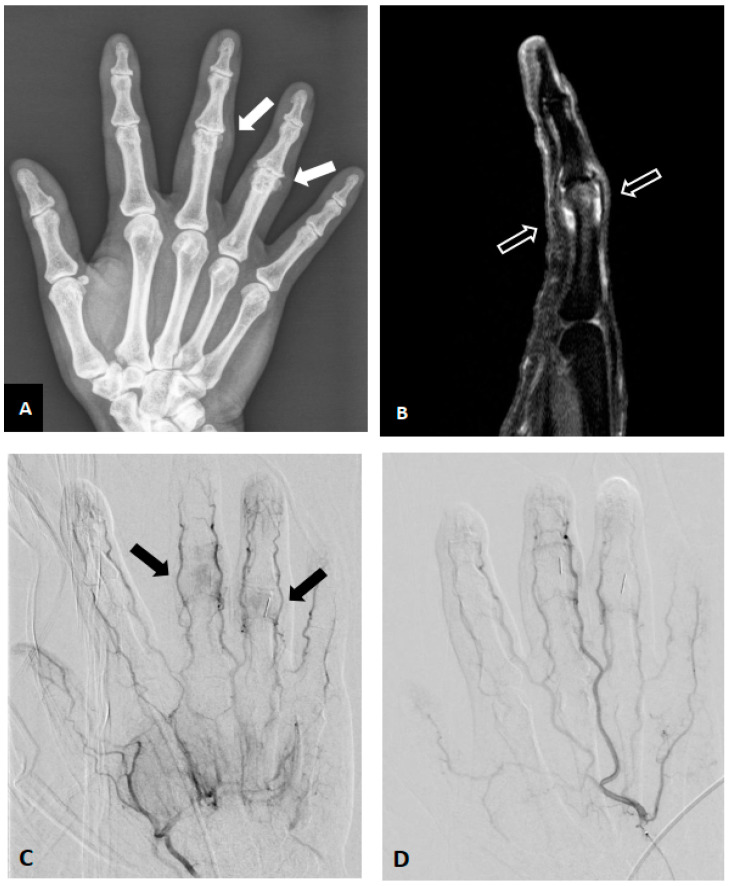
Representative pre- and postprocedural images of the right hand 3rd and 4th proximal interphalangeal (PIP) joint osteoarthritis in a 50-year-old female with chronic pain and swelling. The results of all rheumatoid profile blood tests were within the normal ranges. (A) Right hand plain film revealed joint space narrowing with spurs formation (white arrows). (B) Preprocedural sagittal MRI (fat-saturated T1-weighted post-contrast) of the 3rd finger revealed synovium thickening and enhancement at approximately the 3rd PIP joint (blank arrows). (C) Preprocedural angiography of the right ulnar artery showed angiogenesis (black arrows) overright 3rd and 4th PIP joints. (D) Postembolization angiography via ulnar artery indicated elimination of angiogenesis after embolization. The patient’s joint pain and swelling both were improved after treatment.
